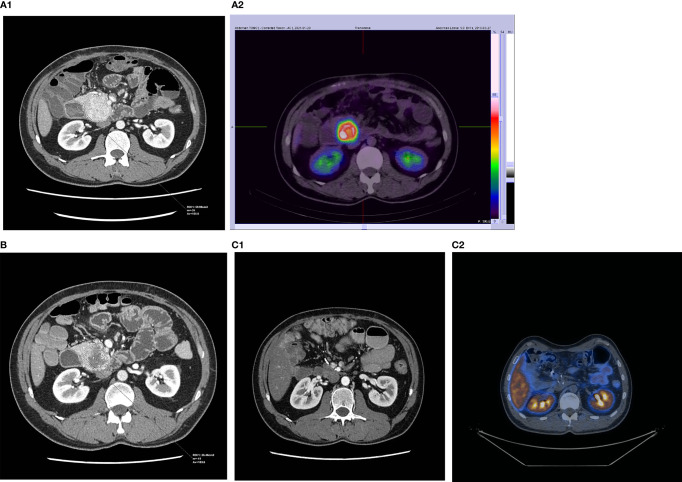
CT and SRI scans of a patient with successfully operated NET of the pancreas: (A1, 2) before PRRT, (B) 3 months after 4 cycles of 177Lu/90Y-DOTA-TATE (only CT), and (C1, 2) after complete tumor removal.
